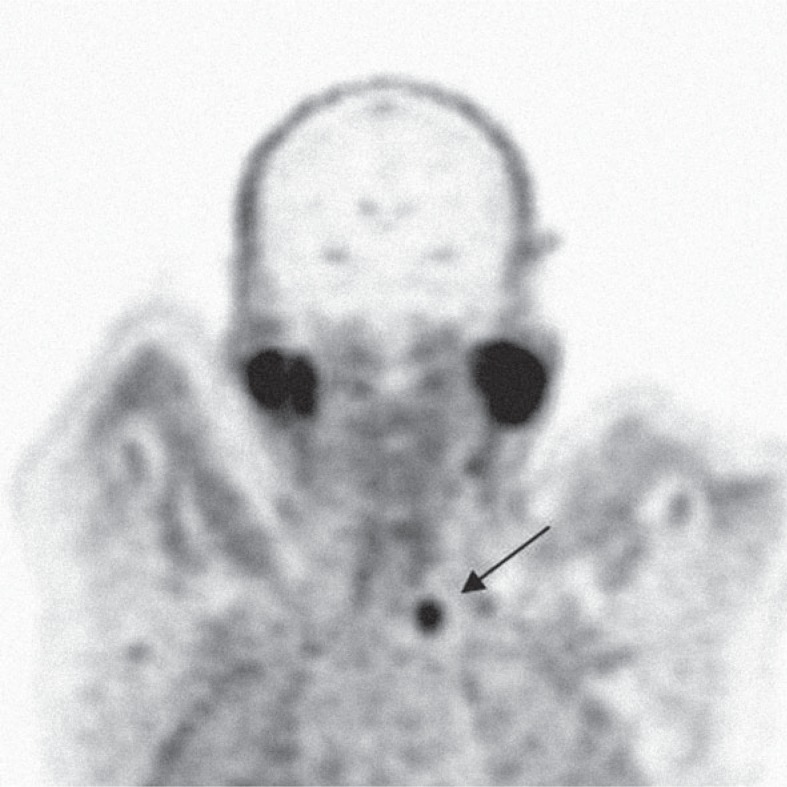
Positron emission tomography-computed tomography coronal slice: Adenoma of the parathyroid that incidentally took-up ^18^F-fluorocholine (SUVmax 3.4).
